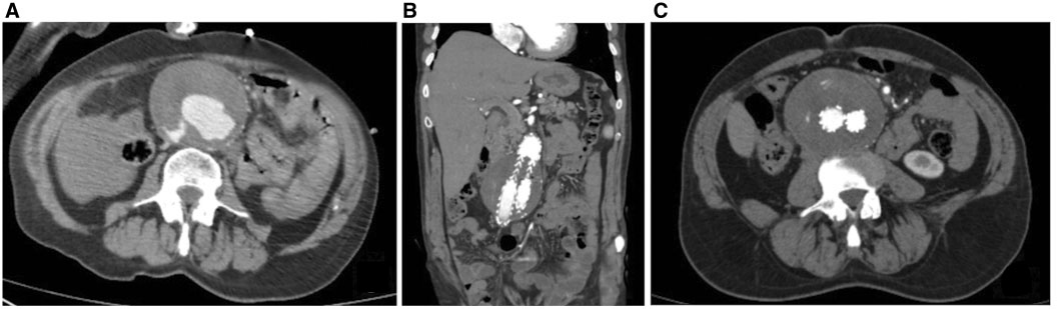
The computed tomography image of an abdominal aortic aneurysm. (A) A computed tomography image of a ruptured abdominal aortic aneurysm. (B) An image of endovascular repair of the aortic aneurysm repair. (C) The endovascular graft could seen in the aortic lumen in the same patient in the follow-up period; no adverse event was detected.
